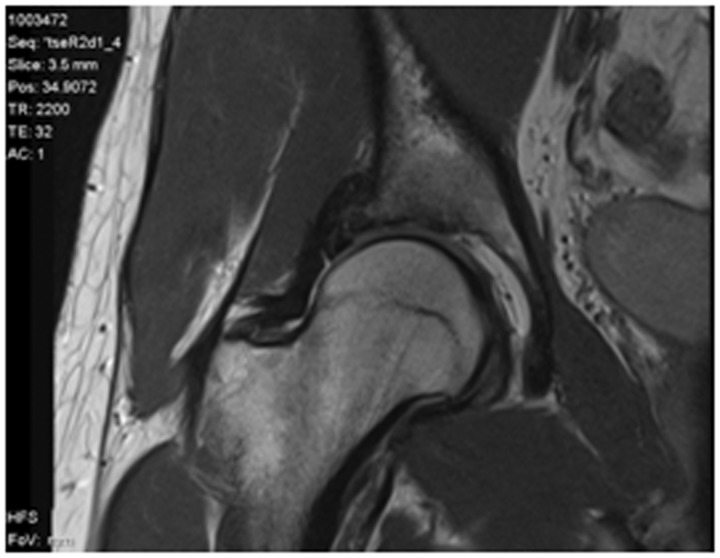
Slice from 3 Tesla MRI scan.
